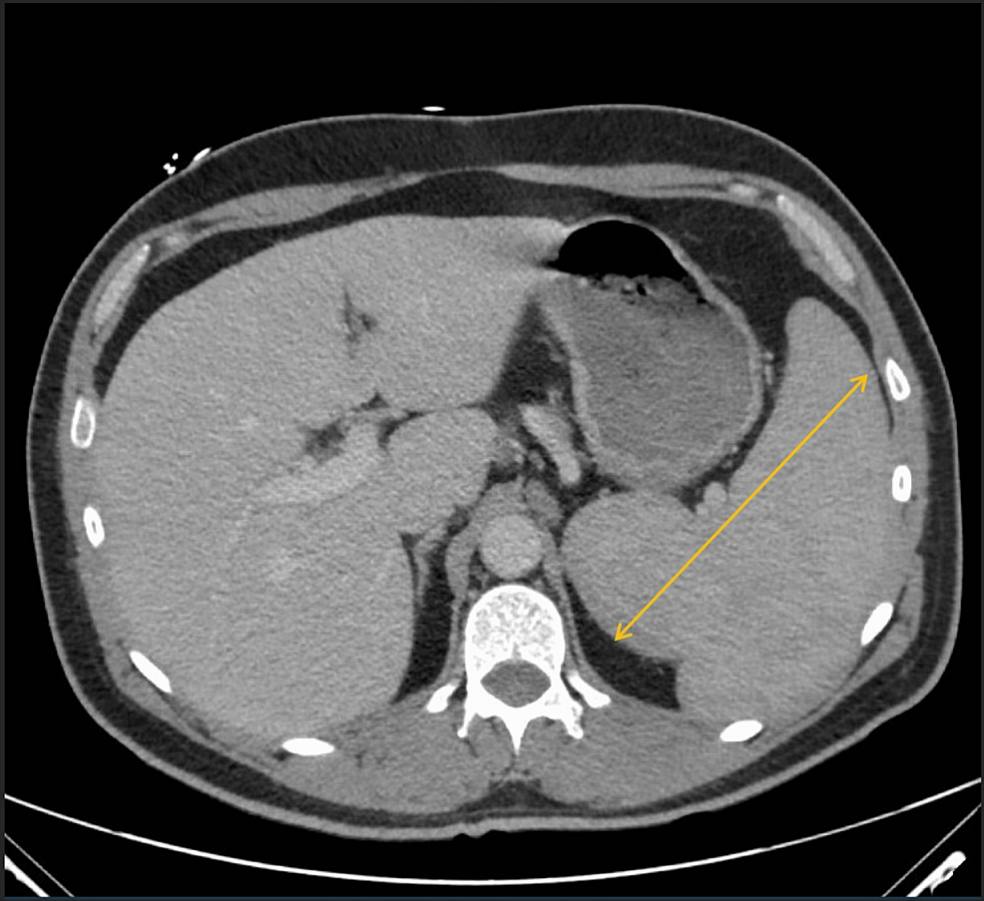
CT scan showing hepato-splenomegaly; yellow line demonstrates splenomegaly
